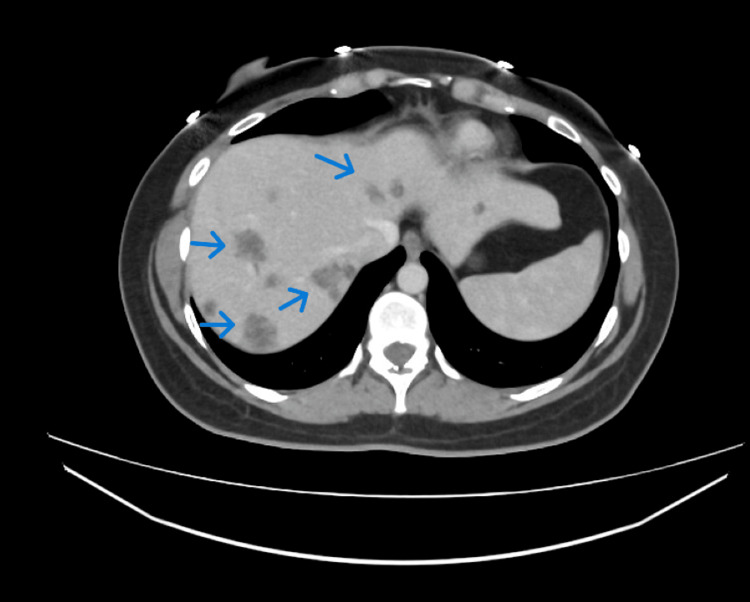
CT abdomen with contrast performed in December 2022 demonstrating multiple liver metastasis (blue arrow)
